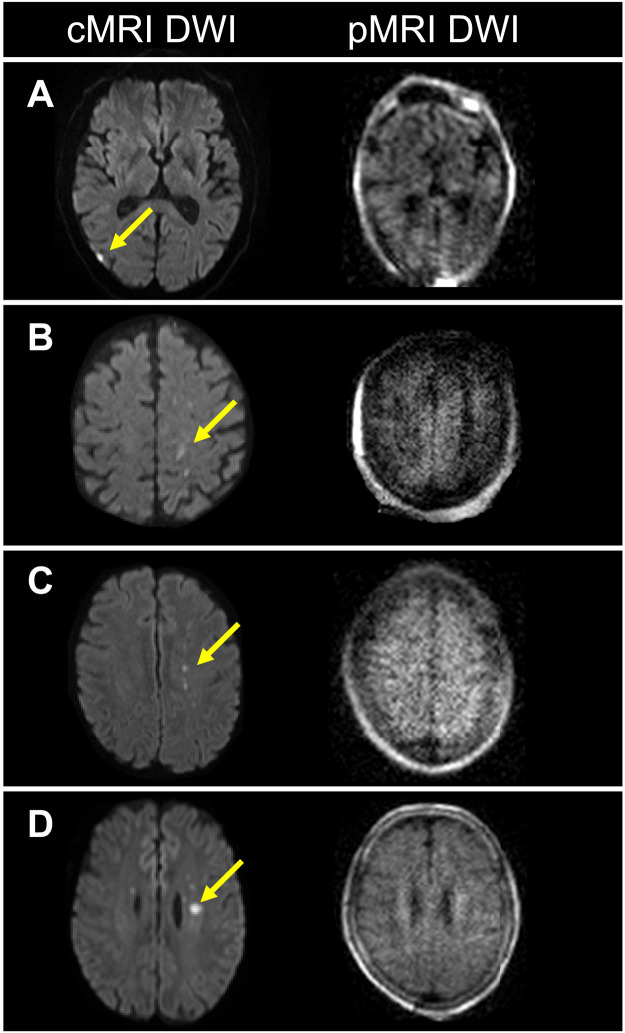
Patients with ischemic stroke with infarcts not visualized by pMRI. Four patients with ischemic stroke (A to D) had foci of restricted diffusion (yellow arrows) that were detected solely by conventional MRI (cMRI) (1.5/3 T) DWI sequences, but not pMRI DWI. (A) 7-mm-diameter infarct. (B) 10-mm infarct. (C) 4-mm infarct. (D) 7-mm infarct.
